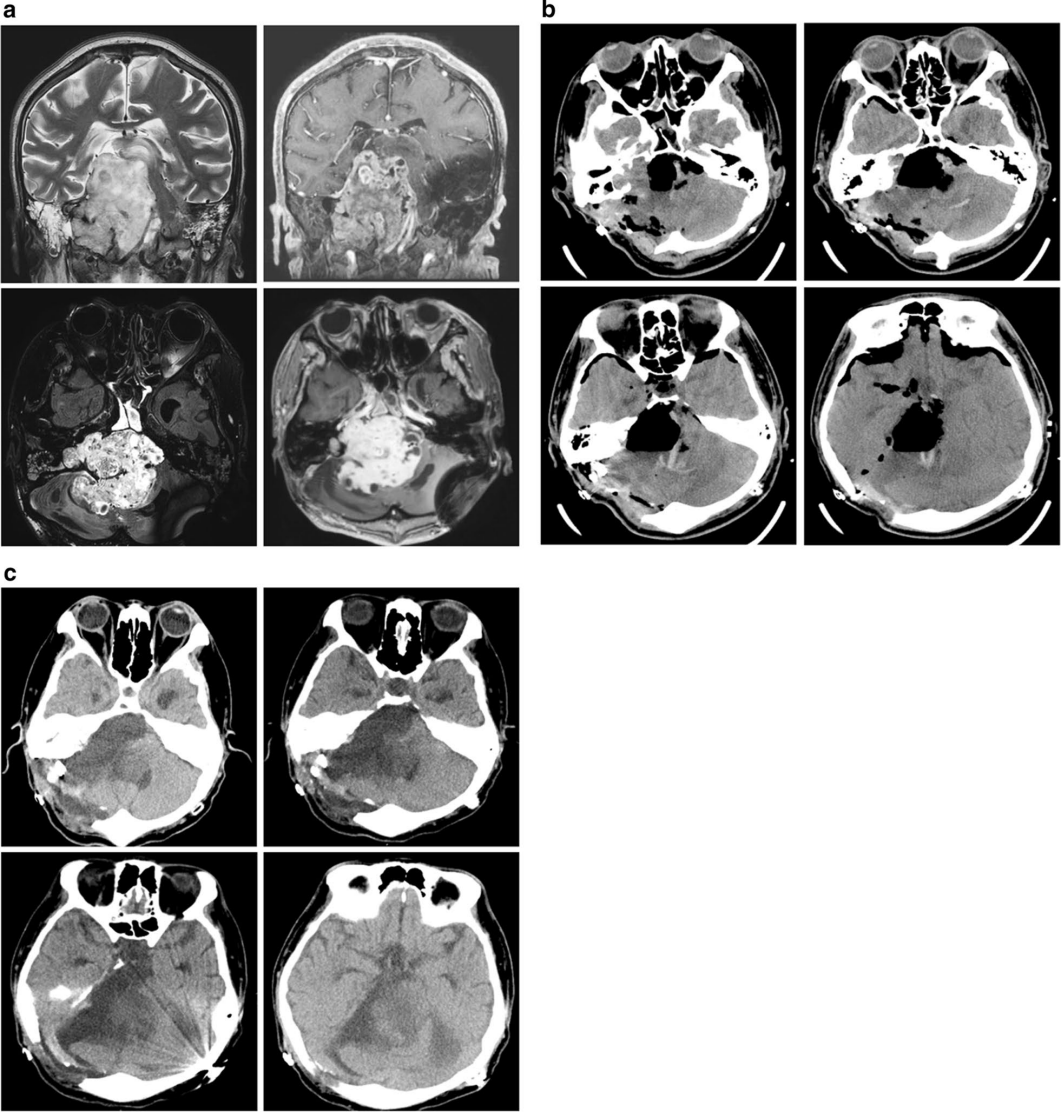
a. Preoperative MRI of patient 2 showing PSH. A 7.7 cm-sized homogenous enhancing cystic and solid extraaxial mass originates form jugular foramen. This mass extends from retroclival area to posterior fossa compressing brainstem. (*Clockwise direction*; T2WI coronal, T1W enhance coronal, T1 W enhance axial, 3D FLAIR). b. Immediate postoperative CT scan demonstrating focal hemorrhage and edema in compressed brainstem. c. Postoperative CT scan (POD 14) when the PSH occurred
